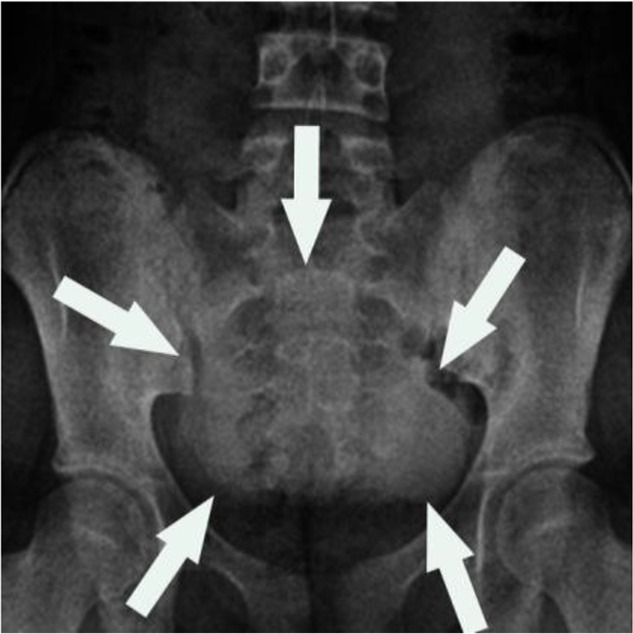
Radioopaque area in the standing direct abdominal radiograph
